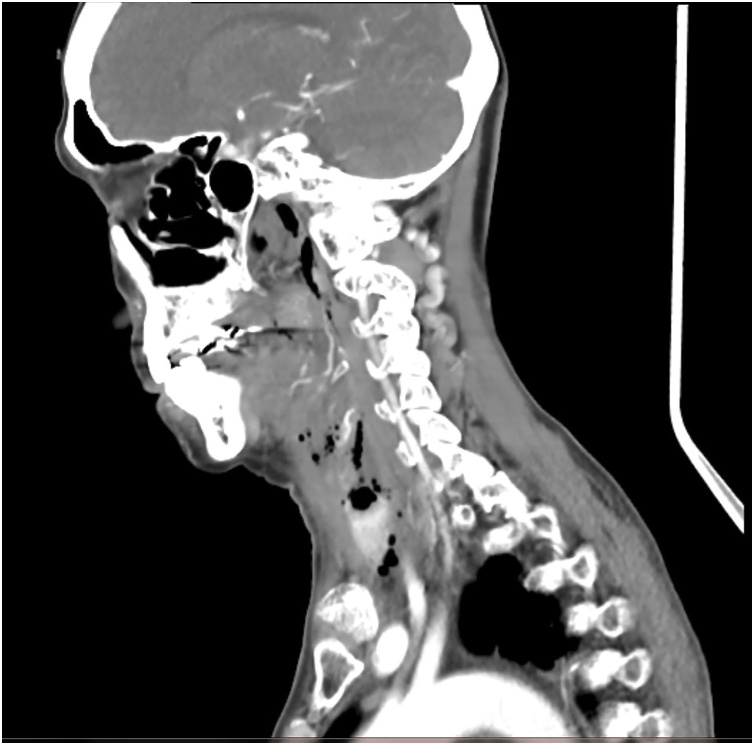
Axial view of plain CT of the neck (post gastrograffin) showing contrast leakage within the previously hypodense collection at the left paravertebral region.
